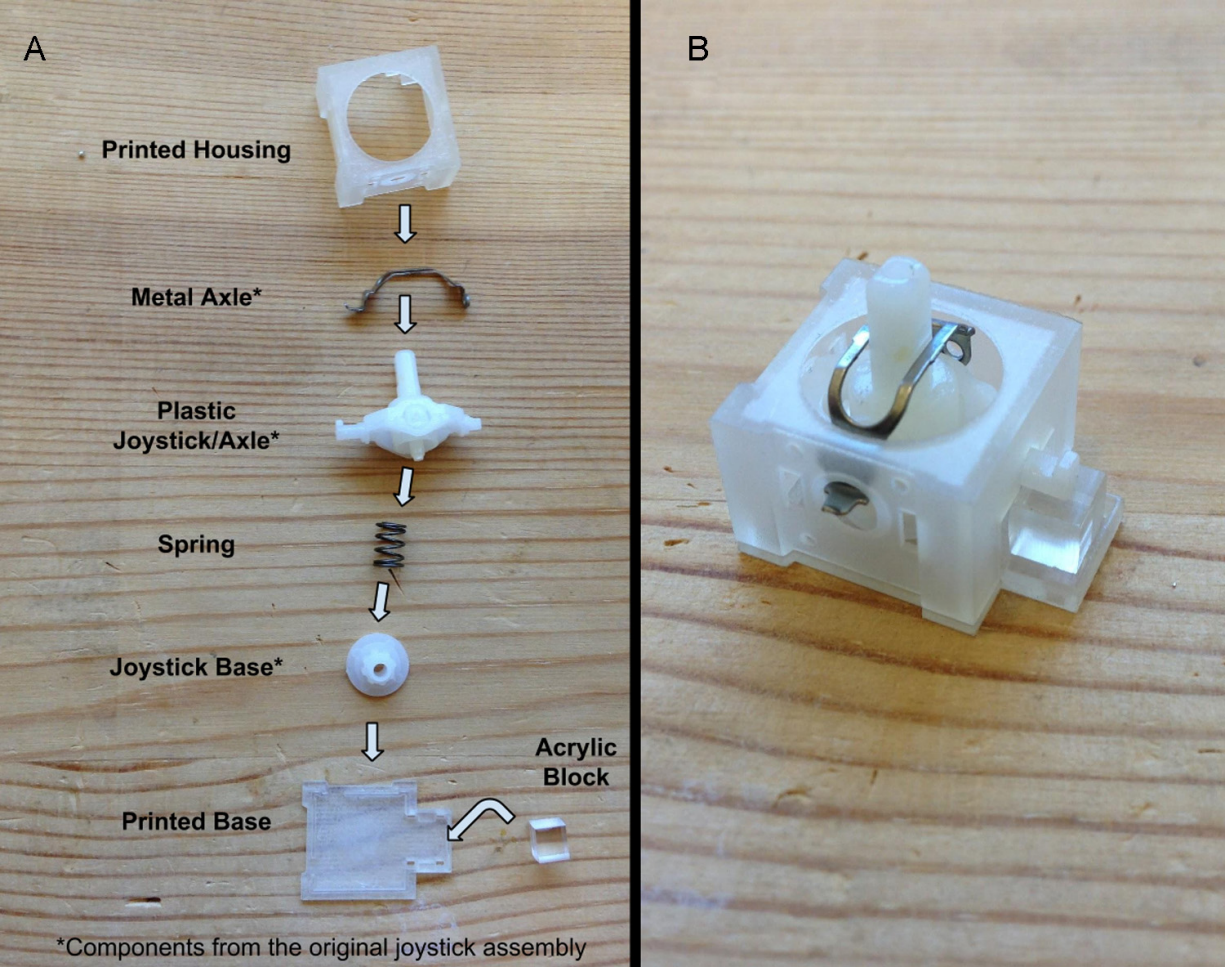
(A) A step-by-step layout of the pieces necessary to reconstruct the joystick assembly. The component names with an asterisk are from the stock joystick assembly and do not need to be made. (B) Fully re-assemble, MRI safe, joystick assembly.
